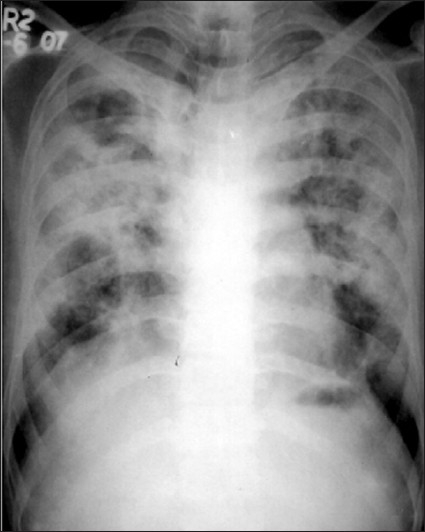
Chest radiograph (PA view) after chest tube removal showing fully expanded lungs with diffuse reticulonodular shadows
